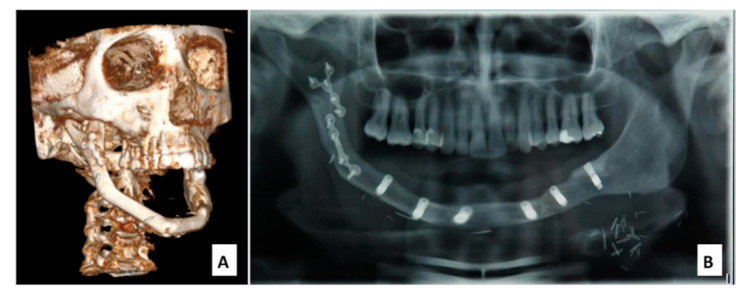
(A) CT scan 3D before prosthetic rehabilitation (B) OPT after placement of dental implant.
